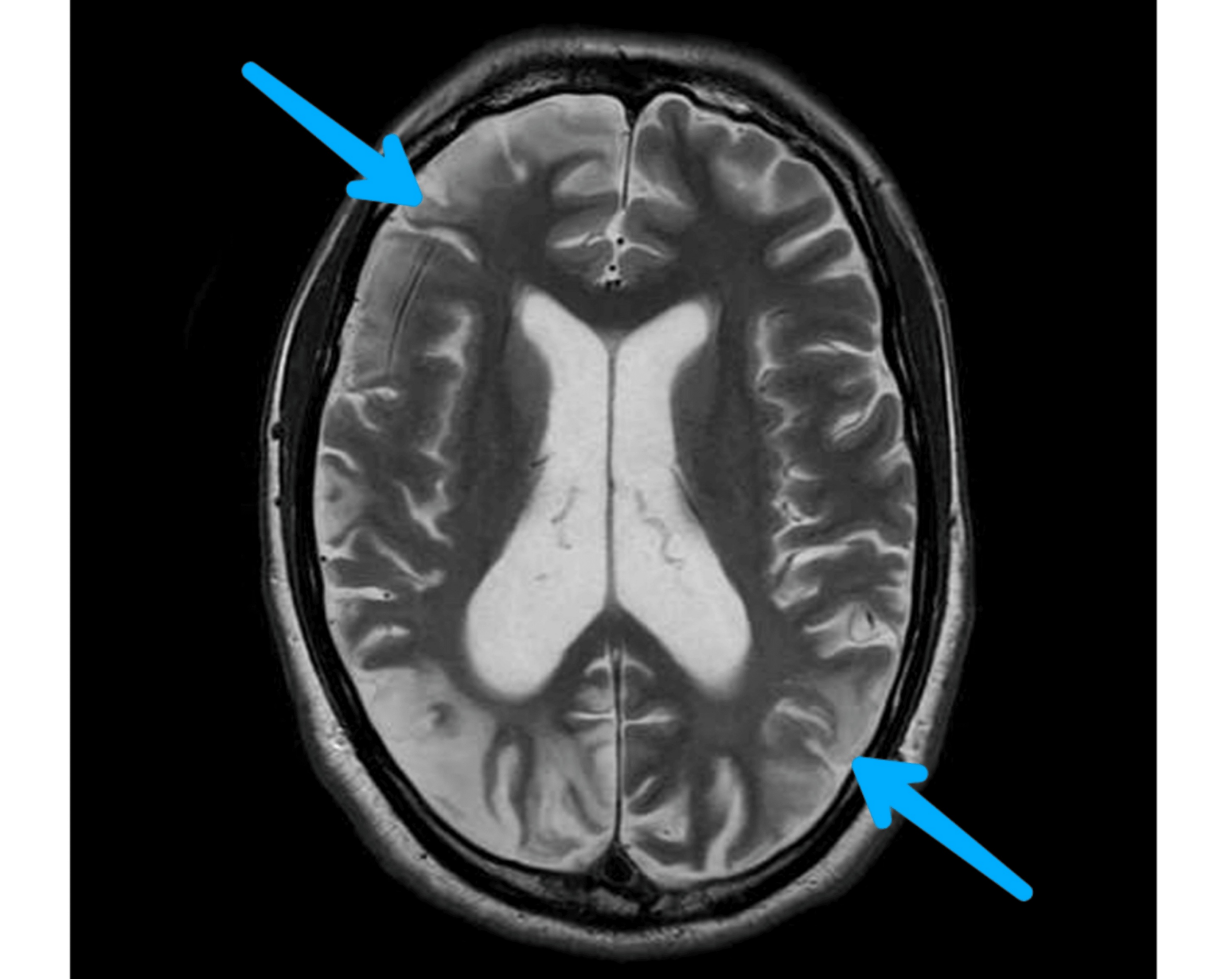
MRI head image after three weeks Interval resolution of infarcts seen in repeat MRI head after three weeks.
